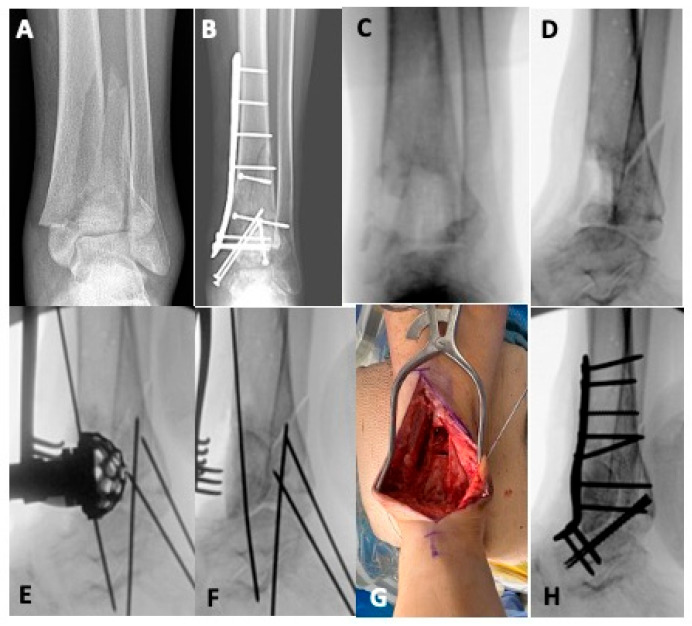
A twenty-four-year-old sustained a pilon fracture in a motor vehicle collision. Initial radiographs (A) demonstrated an AO 43-C fracture with metaphyseal comminution. His course was complicated by infection, and despite multiple attempts at irrigation and debridement with hardware retention at an outside facility. He presented to our institution with an infected nonunion and draining sinus (B) at six months after his original injury. He was managed in a staged fashion. After removal of hardware and adequate debridement of nonviable bone and prior bone graft, he had a large anterior defect (C,D) that was temporized with antibiotic-impregnated cement. After infection clearance, he was managed definitively with tibiotalar arthrodesis using autologous cancellous bone graft and structural femoral head allograft to fill the bone void. An acetabular reamer was used to prepare the graft bed (E), then a femoral head allograft was appropriately fashioned to fill the defect (F,G), followed by a compression plate and screw construct for tibiotalar arthrodesis (H).
